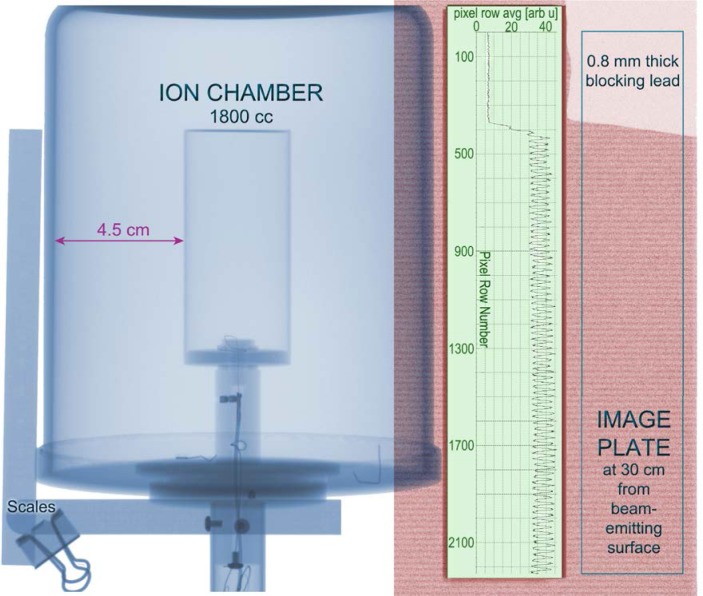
X-Ray image of a large-area ion chamber (left) proportionately scaled and superimposed upon an image acquired 30 cm from the beam-emitting surface of an AS&E SmartCheck X-ray Inspection System that employed an x-ray tube potential of 100 kV. External (clamped) scales are seen, establishing the distance between the cylindrical electrodes. The right image shows the relative signal of the rastered pencil beam of x rays as registered by an image plate detector; the upper right hand corner was blocked by lead to determine the background-signal level of the image plate. The vertical spatial variation in signal is plotted by averaging the pixel values in each row for the tall rectangular region of interest shown.
